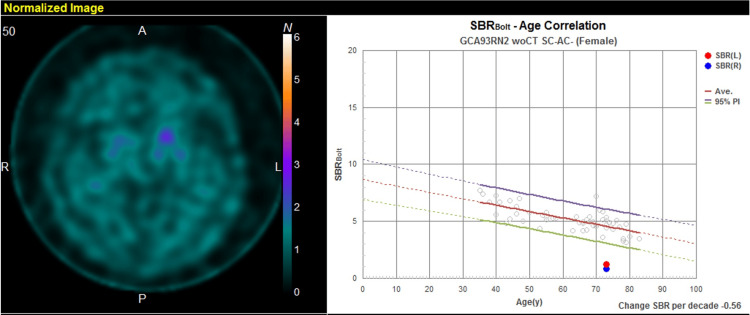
Dopamine transporter single-photon emission computed tomography (DaT-SPECT) with 123I-Ioflupane findings. DaT-SPECT demonstrated decreased accumulation predominantly on the right side (striatal binding ratio {SBR}: right 1.41, left 2.34).
